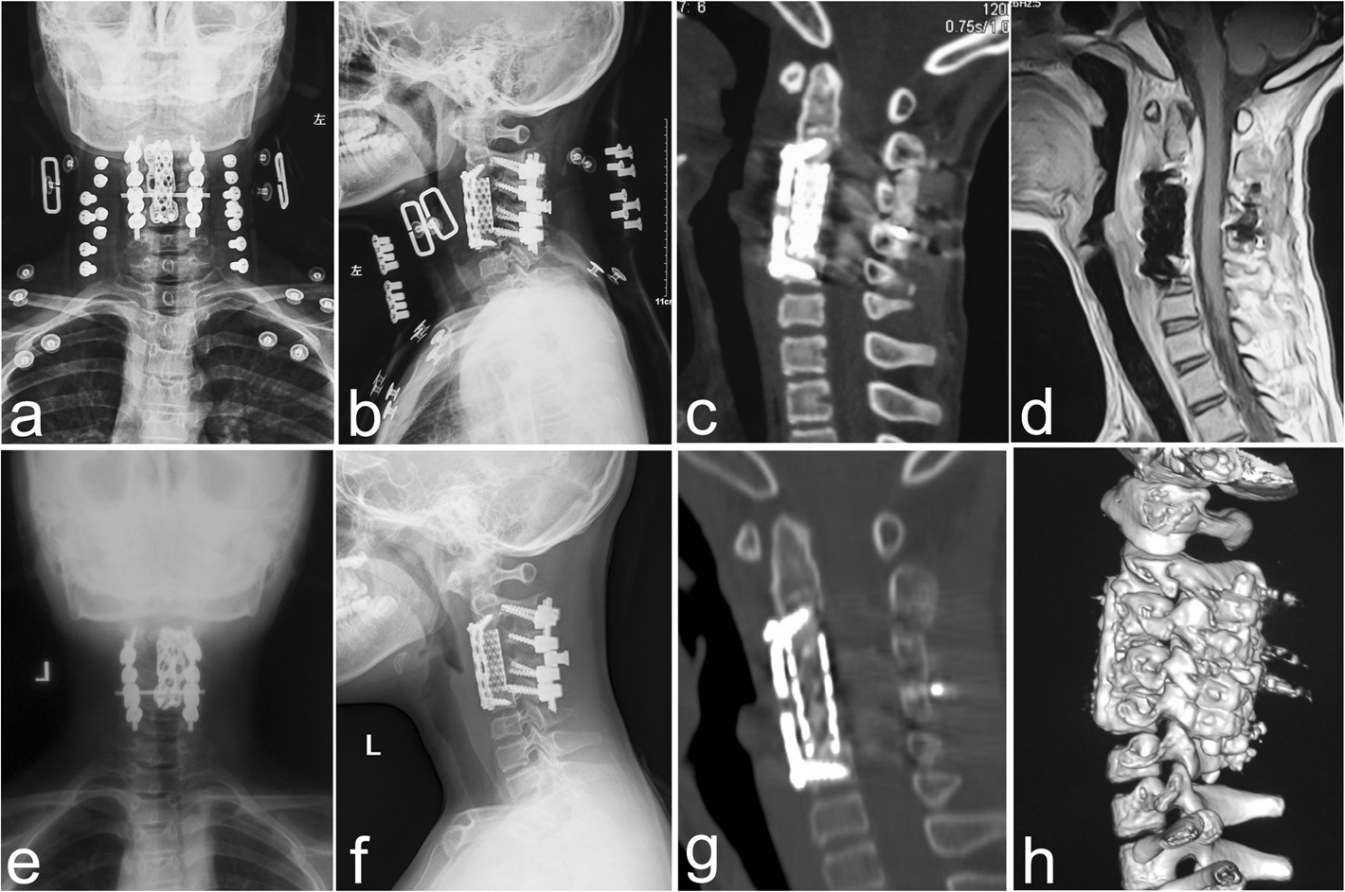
Postoperative and follow-up images of patient no. 5 are shown. (a and b) Postoperative anterior-posterior and lateral X-ray indicate that the kyphosis significantly improved using one-stage 540-degree fusion combined with anterior debridement and decompression. Sagittal CT (c) and MRI (d) revealed satisfactory focal clearance and decompression without graft and instrumentation-related complications and relapse of Pott’s disease at three months postoperation. (e and f) Anterior-posterior and lateral X-ray revealed that the screws, rods, plate and TMC was in good shape without fractures and displacement at 12 months postoperation. Sagittal CT-scan (g) and three-dimensional reconstruction (h) revealed continuous bridging, and the bone trabeculae at the graft-host vertebral endplate junction had no pseudoarthrosis formation; indicating the superb maintenance of correction of kyphosis at the final follow-up
